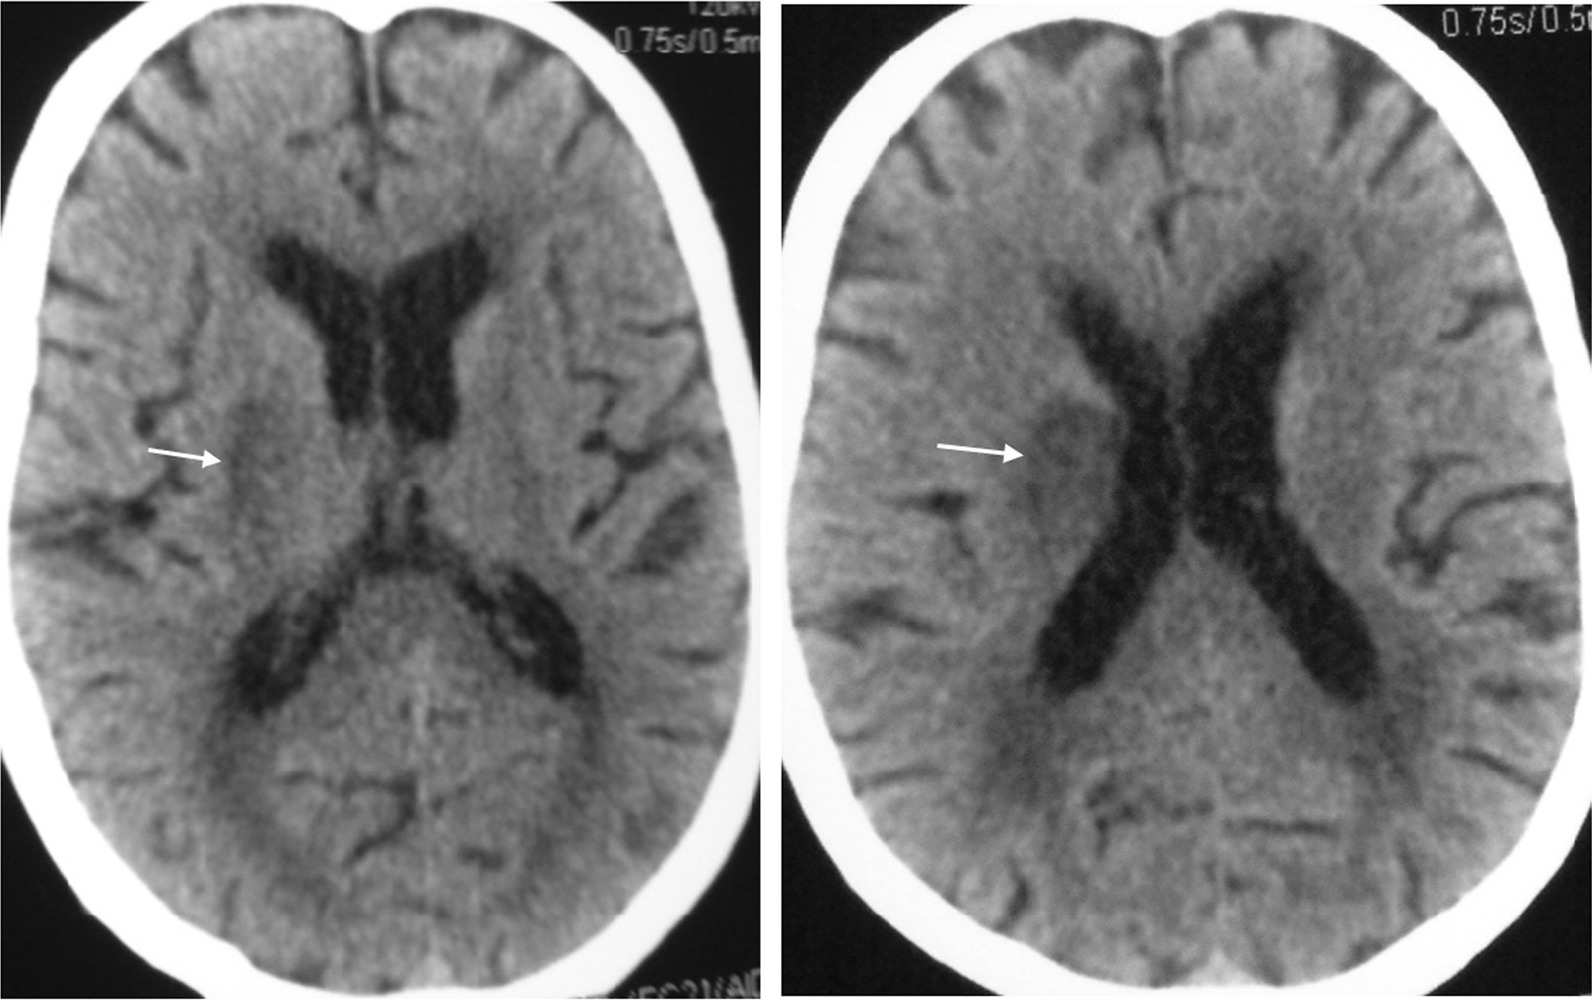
Computed tomography scan of brain showing an ischemic infarct in right-sided internal capsule (arrow)
